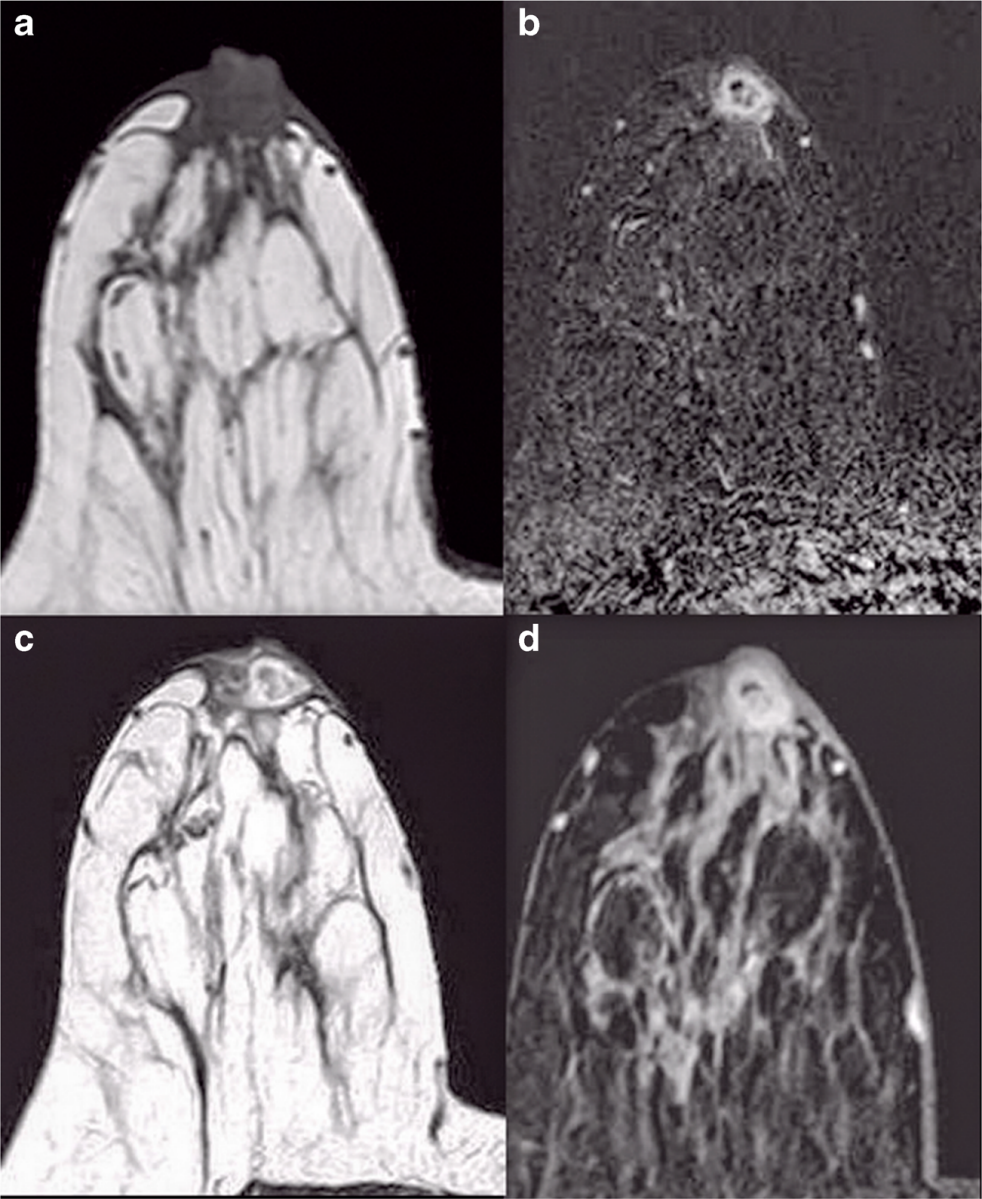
MRI of the right breast of the same patient as in Fig. 12 shows an irregular T1W isointense (a) lesion that is hyperintense on STIR (b) and T2W (c) with central hypointense area and peripheral enhancement on post-contrast T1W sequence. Biopsy from the nipple areolar region was suggestive of chronic sub-areolar abscess. The patient improved on oral antibiotics
